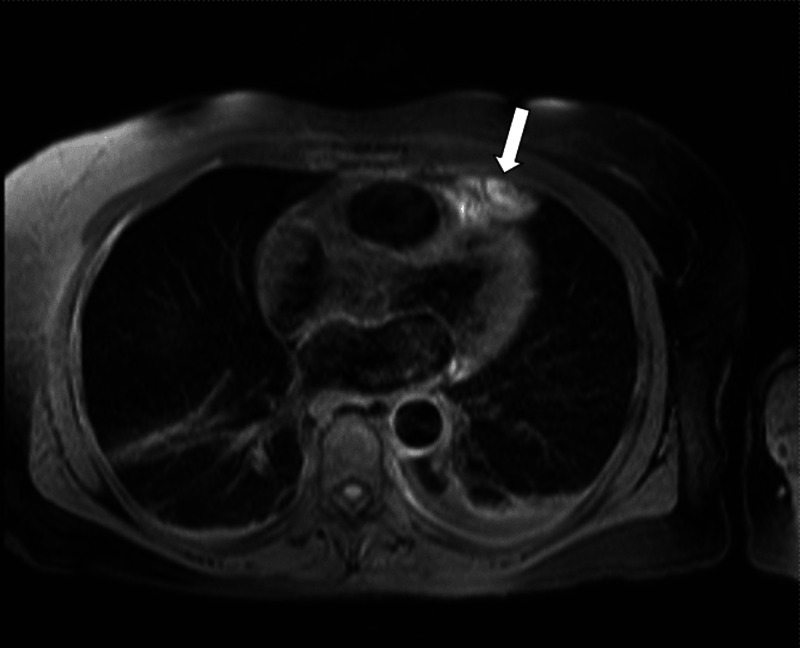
Magnetic resonance image T2-weighted high-intensity signal suggestive of an active lesion of epicardial fat necrosis as indicated by an arrow. Image provided by the Beaumont Hospital, Royal Oak with permission for use.
